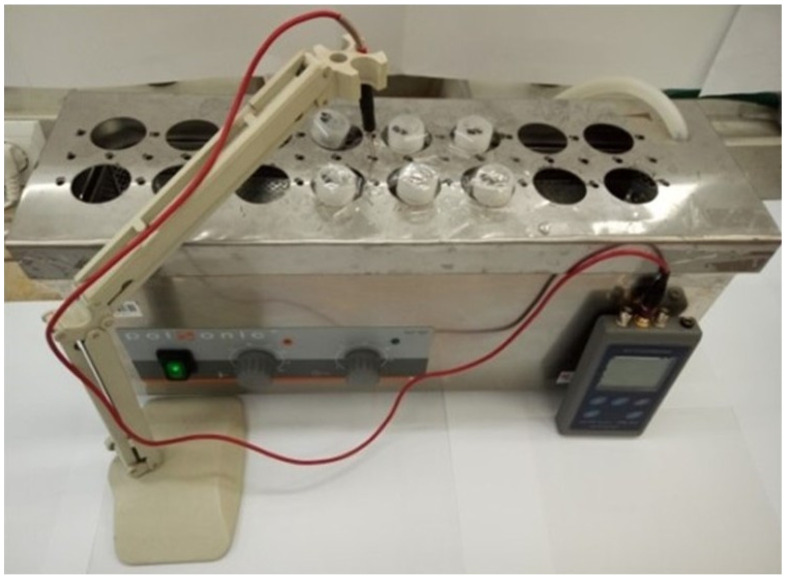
Laboratory-scale setup for the ultrasound treatment, equipped with temperature and time controllers.
